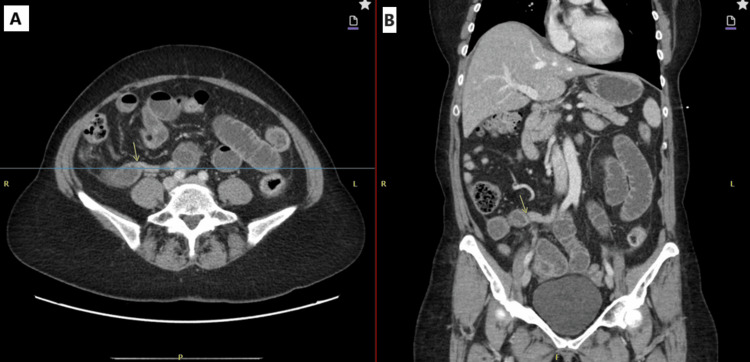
CT abdomen/pelvis: (A) axial and (B) coronal views CT: computed tomography
